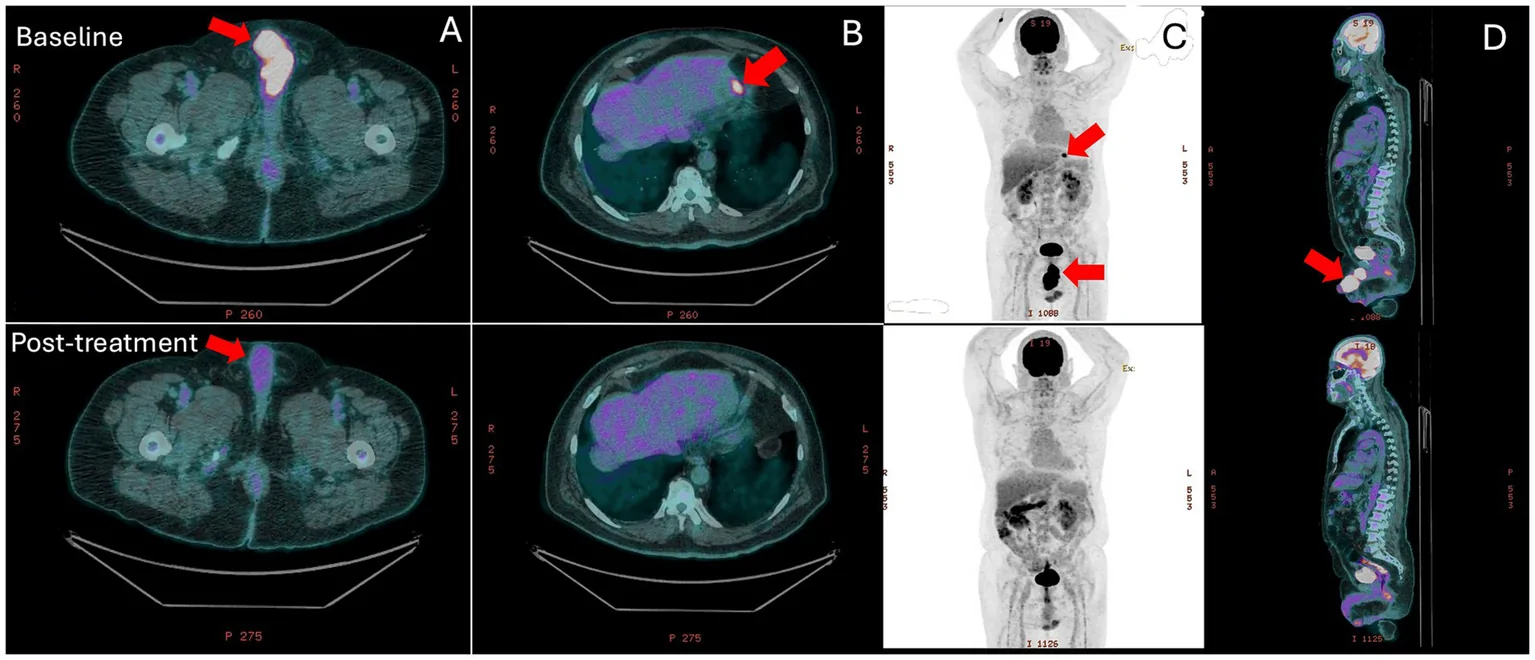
Comparison of baseline and post-treatment FDG PET/CT findings. Baseline images (upper row) and follow-up images after the fourth cycle of R-CHOP therapy (lower row) are shown: (A) Axial fused PET/CT images demonstrate intense FDG uptake in the penile lesion at baseline (SUVmax 34.28 early and 36.76 delayed), which markedly decreased on follow-up (SUVmax 2.95), consistent with significant metabolic response (Deauville score 5 → 3). (B) Axial fused PET/CT images of the stomach show an additional FDG-avid lesion at baseline (SUVmax 6.82 early and 7.96 delayed), with markedly reduced uptake after treatment (SUVmax 2.69–3.23), indicating interval metabolic regression (Deauville score 3 → 2). (C) Whole-body maximum intensity projection images demonstrate focal FDG uptake in the penis and stomach at baseline, with marked reduction of previously noted hypermetabolic lesions and no newly identified FDG-avid lesions on follow-up. (D) Sagittal fused PET/CT images show intense FDG uptake in the penile mass at baseline, with markedly decreased metabolic activity after treatment, consistent with treatment response.
